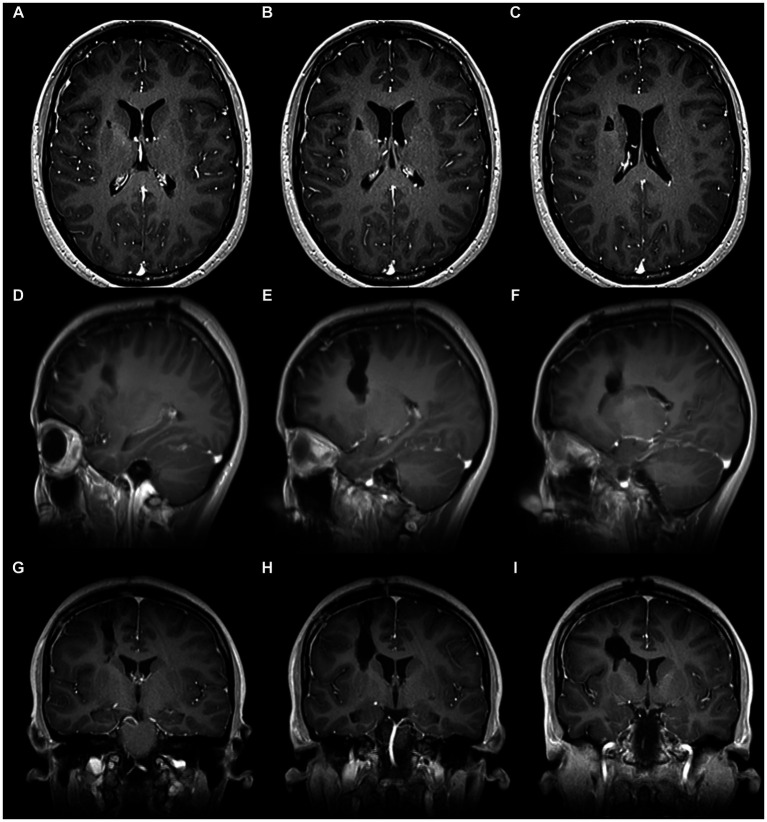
Postoperative brain MRI of cavernoma of the anterior limb of the internal capsule. (A–C) Axial view. (D–F) Sagittal view. (G–I) Coronal view. It shows the resection of the lesion in the depth of the central core and anterior limb of the internal capsule. Note the trajectory from the cerebral surface to the depth, which indicates a dissection through a safe anatomical plane with minimal involvement of noble structures.
